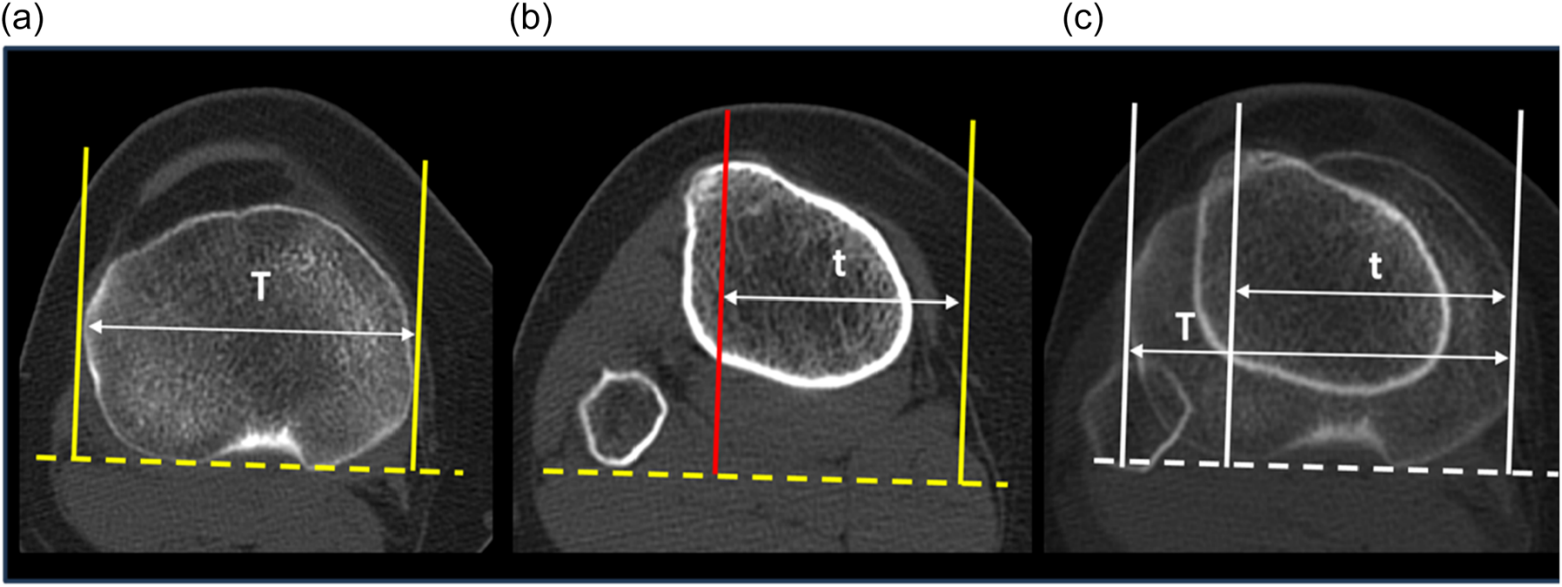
TT lateralisation measurement. (a) Axial CT image of the tibial plateau. The width of the tibial plateau (distance T) is the distance between the two lines perpendicular to the posterior bicondylar line passing through the medial and lateral border of the tibial plateau. (b) Axial CT image of the TT. The distance between the centre of the TT and the medial border of the proximal tibial condyle (distance t) was measured. TT lateralisation was calculated as t ÷ T. (c). Addition CT image. CT, computed tomography; TT, tibial tubercle.
